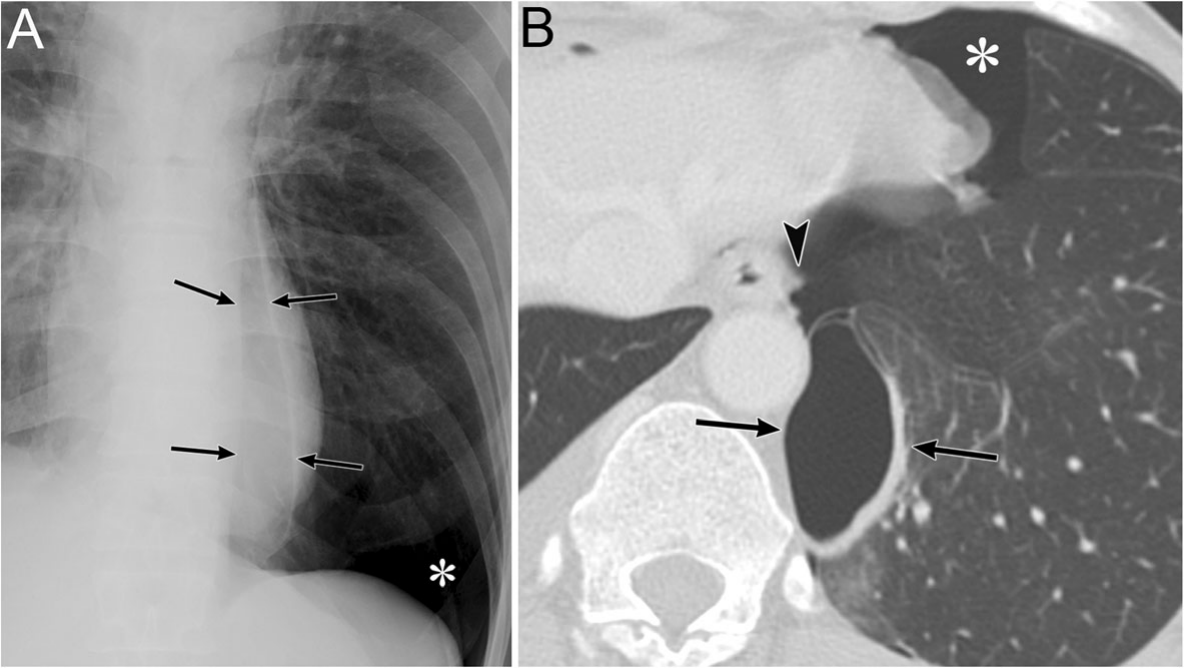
A pneumatocele mimicking inferior pulmonary ligament (IPL) air collection of a 61-year-old man. He has right main bronchial stricture treated under positive pressure ventilation. a A supine radiograph shows an oval lucency (black arrows), which represents a left lower lobe pneumatocele, in the left paramediastinal area. A pneumothorax (white asterisk) is also visible, but the pneumomediastinum is not present. b An axial CT image reveals a left lower lobe pneumatocele (black arrows), while the normal left IPL (black arrowhead) lies along the esophagus. A pneumothorax (white asterisk) is also visible. The pneumatocele and pneumothorax disappeared on the supine radiograph 3 months later
